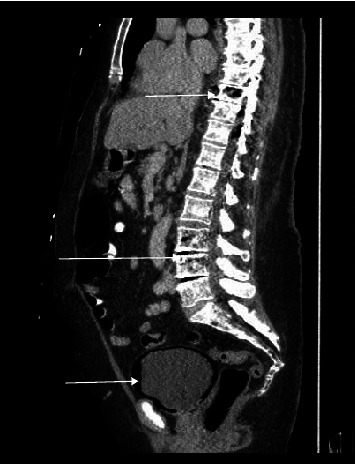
Saggital section on CT showing emphysematous cystitis and pneumoracchis.
